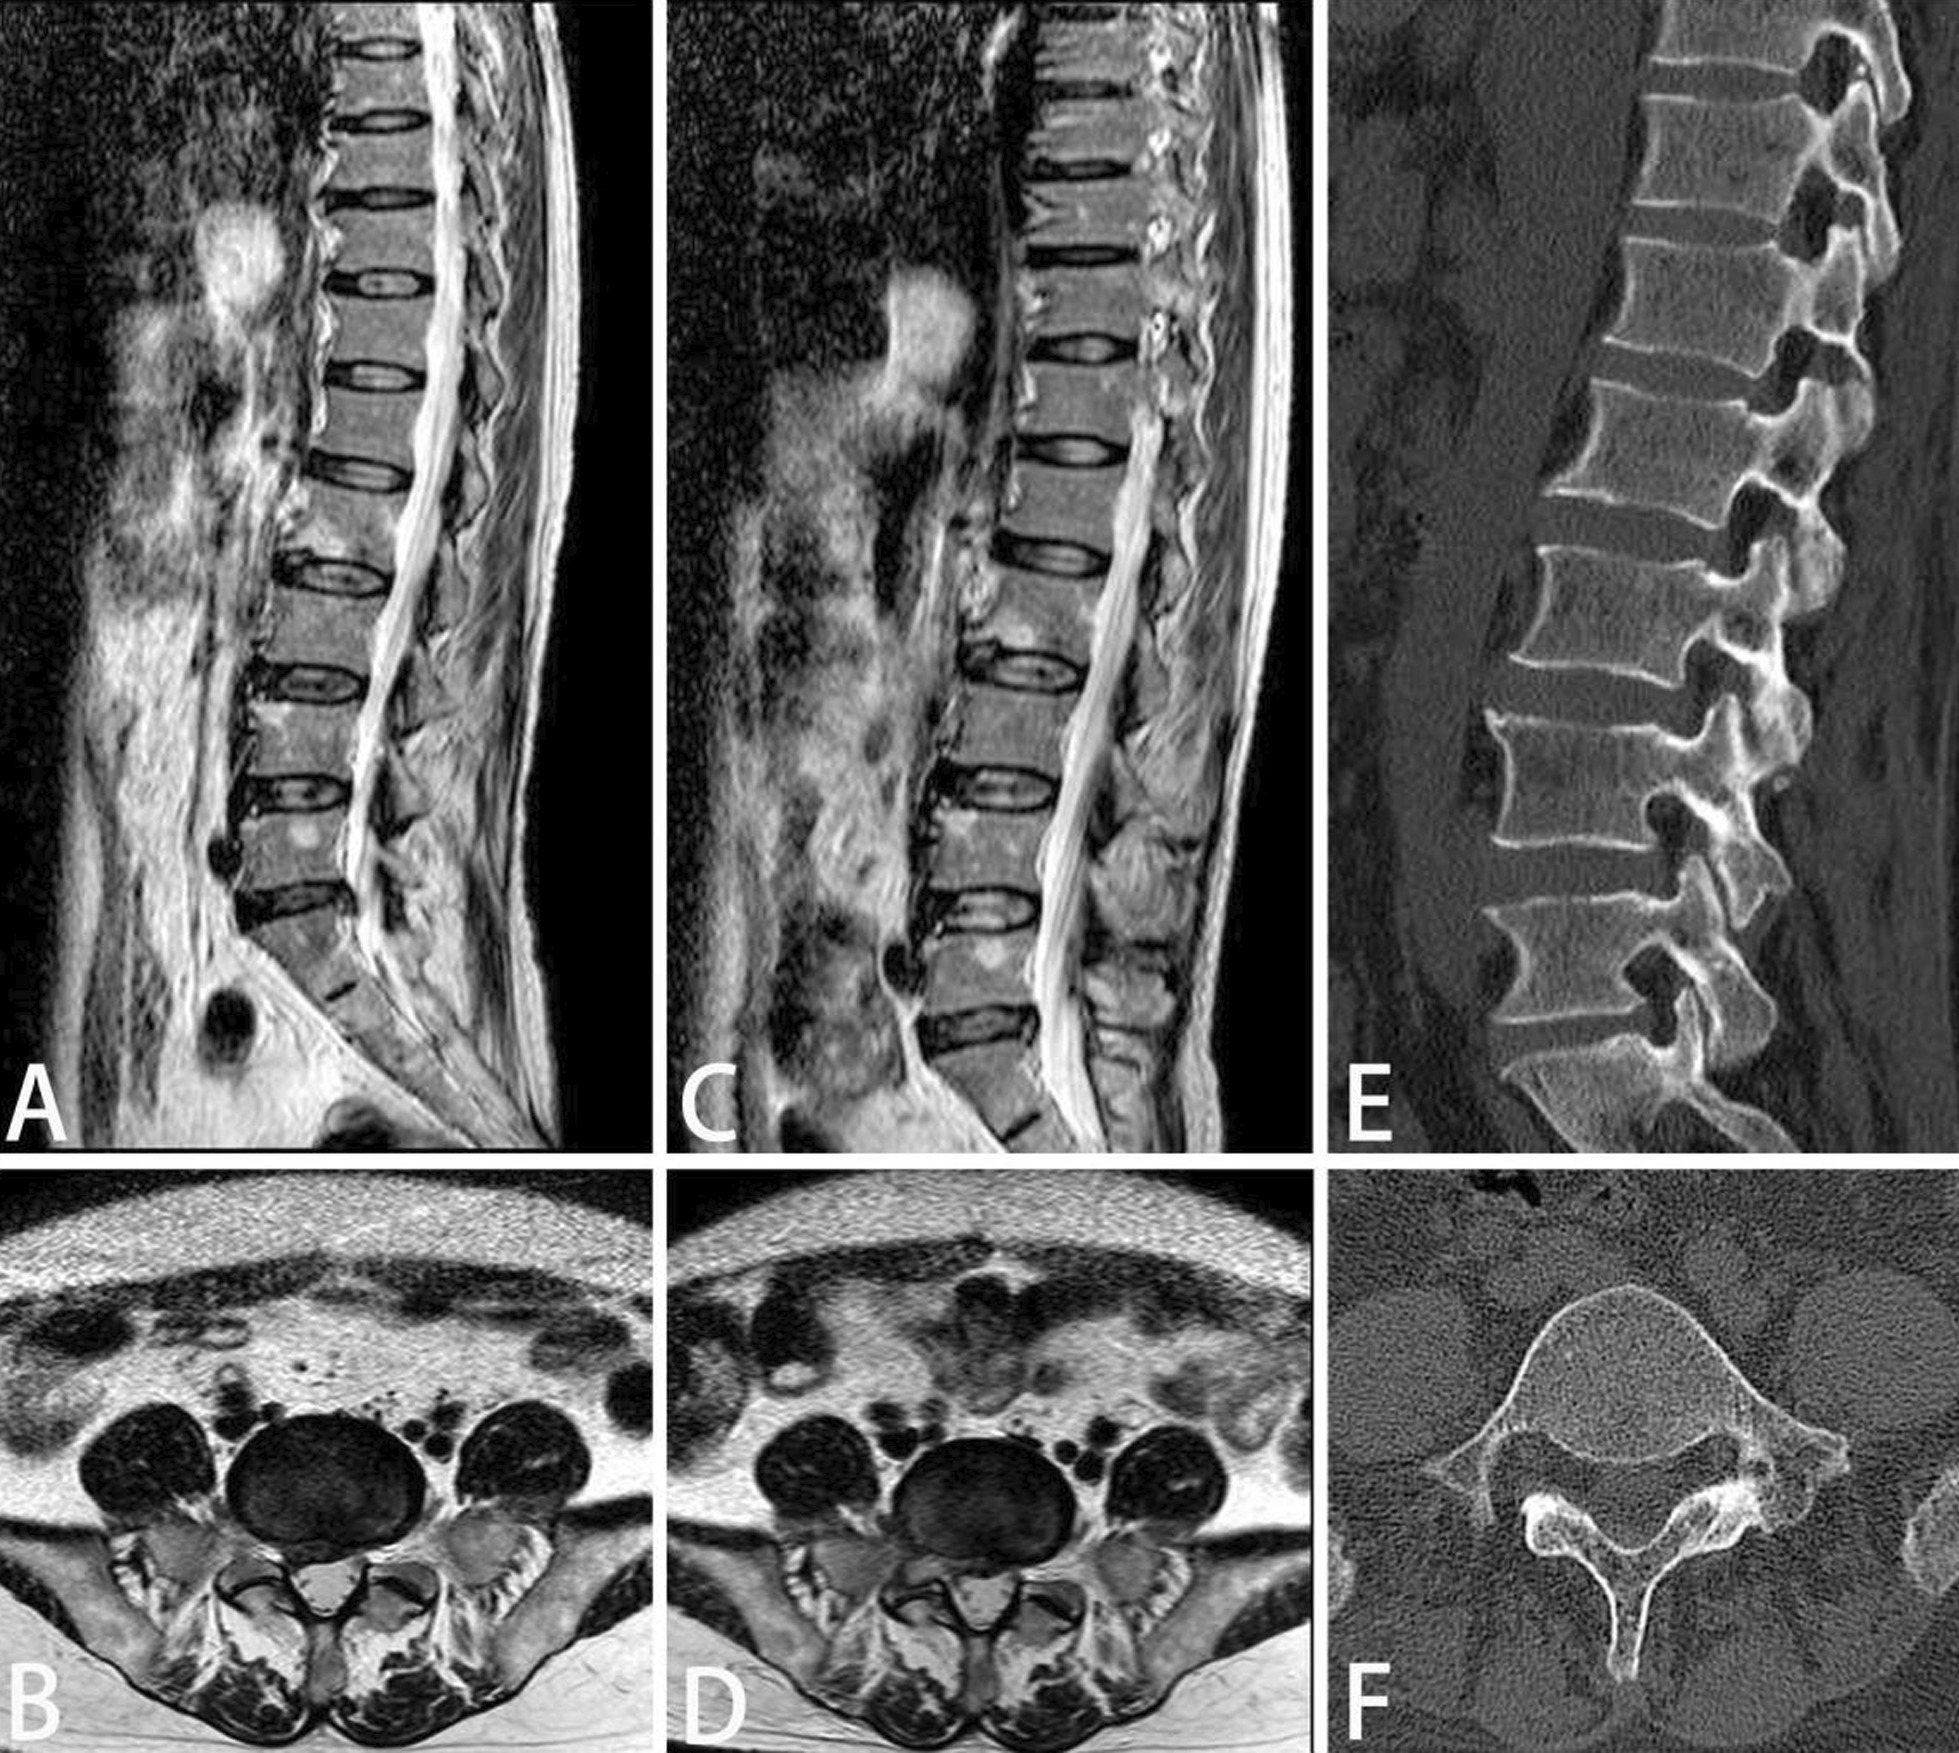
A 64-year-old male patient was diagnosed as type B2 by modified classification. The nucleus pulposus was removed through lateral approach. A–B: Right prolapsed nucleus pulposus was seen on preoperative MRI. C–D: Postoperative MRI showed that the nucleus pulposus was completely removed. E–F: postoperative vertebral CT showed partial bone defects in the foraminal area
